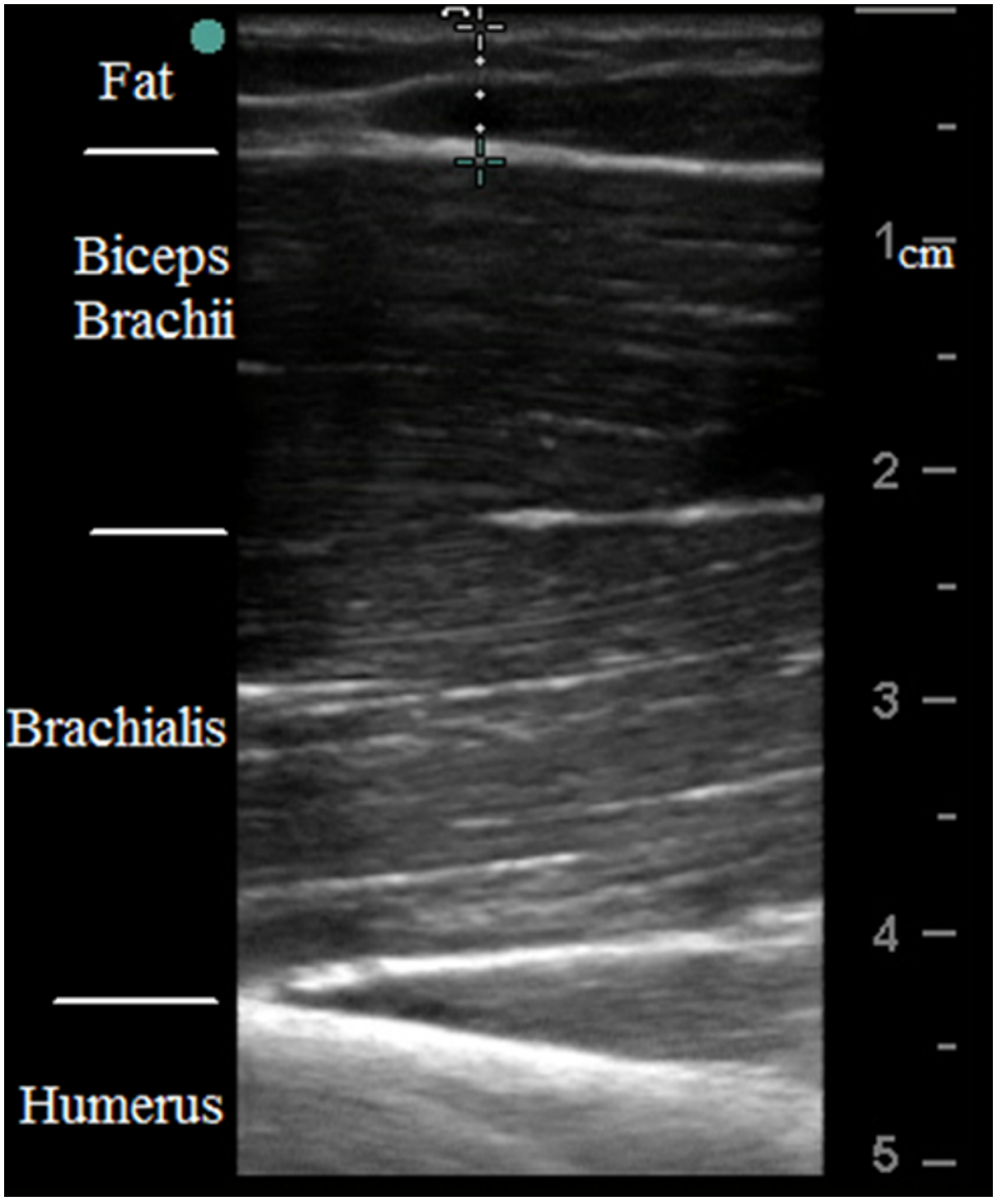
An example of ultrasound data showing the measurement of the SFL thickness.
